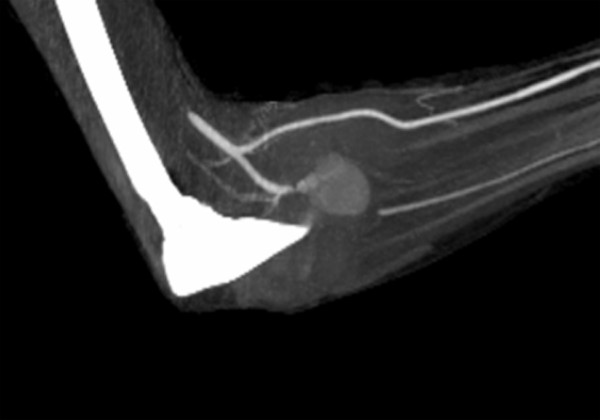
Angio-CT-Scan. False aneurysm of the proximal portion of the interosseous artery (IA).
